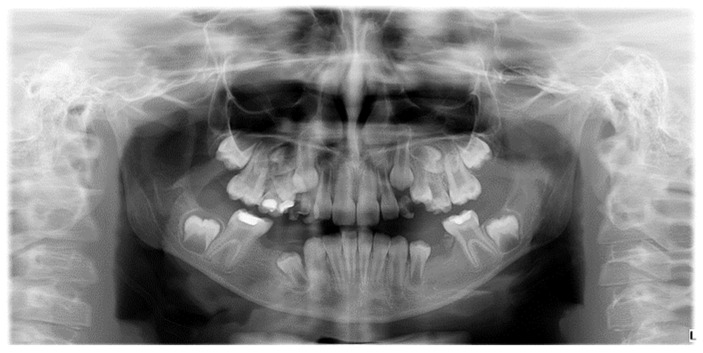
Orthopantomogram of a patient with mixed dentition, clinical diagnosis of MIH, and radiographically certified hypodontia.
